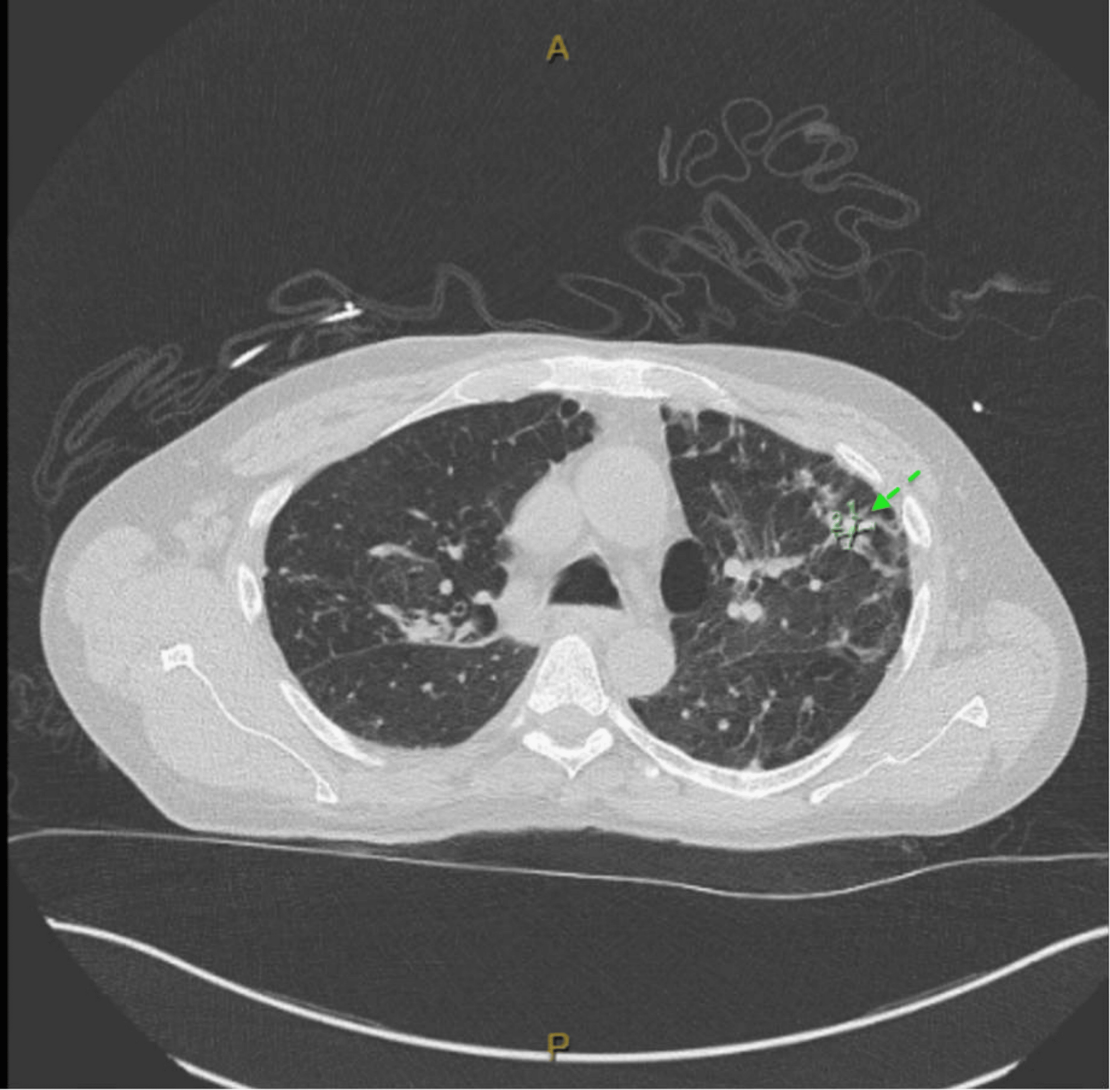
Chest CT showing evidence of tree-in-bud opacities concerning for pneumonia
